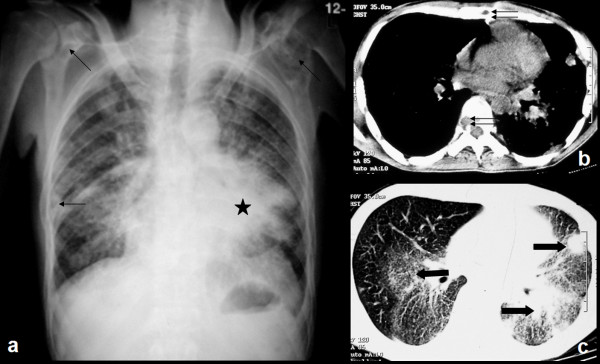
Chest radiograph showing an ill-defined mass in the left mid and lower zones (star), alongwith multiple patches of consolidation in the right lung. Multiple lytic bony lesions can also be seen (arrows). Computed tomography (CT) images (b, c) show the lung masses in detail (solid arrows). Also note the lytic lesions in the sternum and the vertebra (double arrows).
